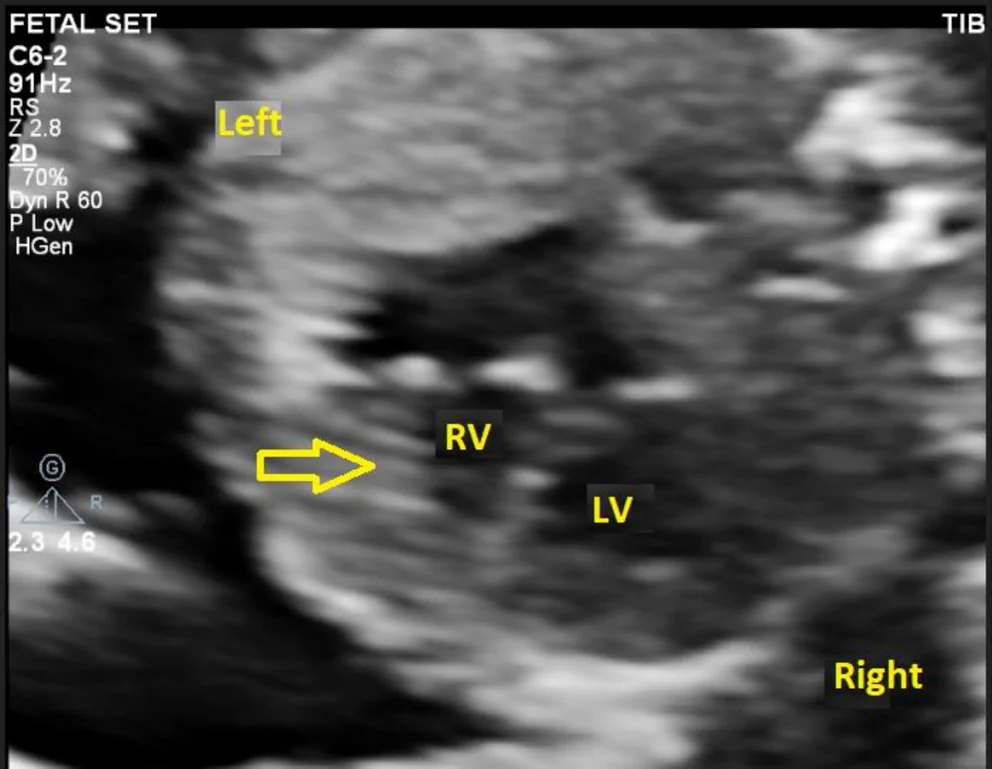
Axial echocardiographic view demonstrates dextrocardia with ventricular inversion and right ventricular free wall hypertrophy (yellow arrow).
